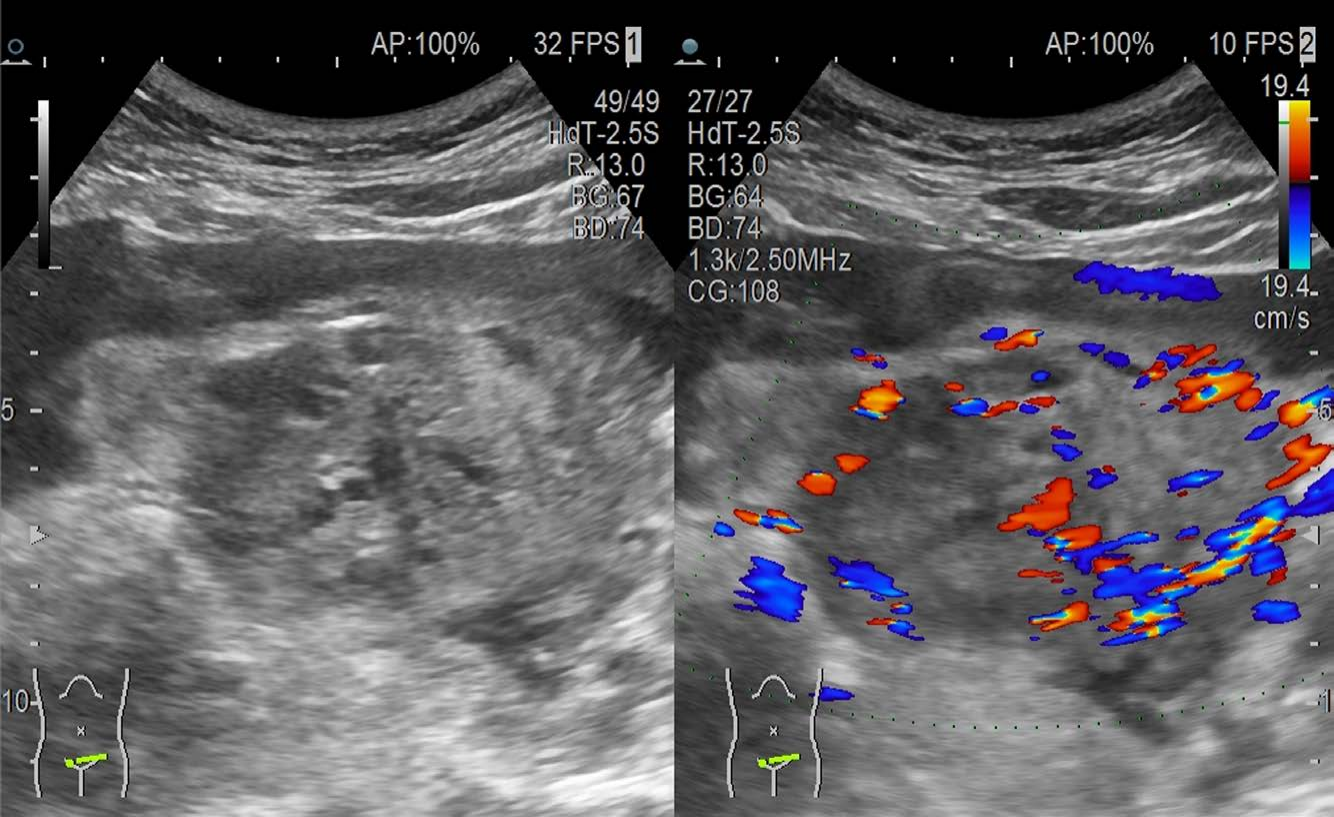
Ultrasound: Mixed mass on the right side of uterus, considering the possibility of ectopic pregnancy rupture, massive fluid accumulation in the pelvic cavity.
